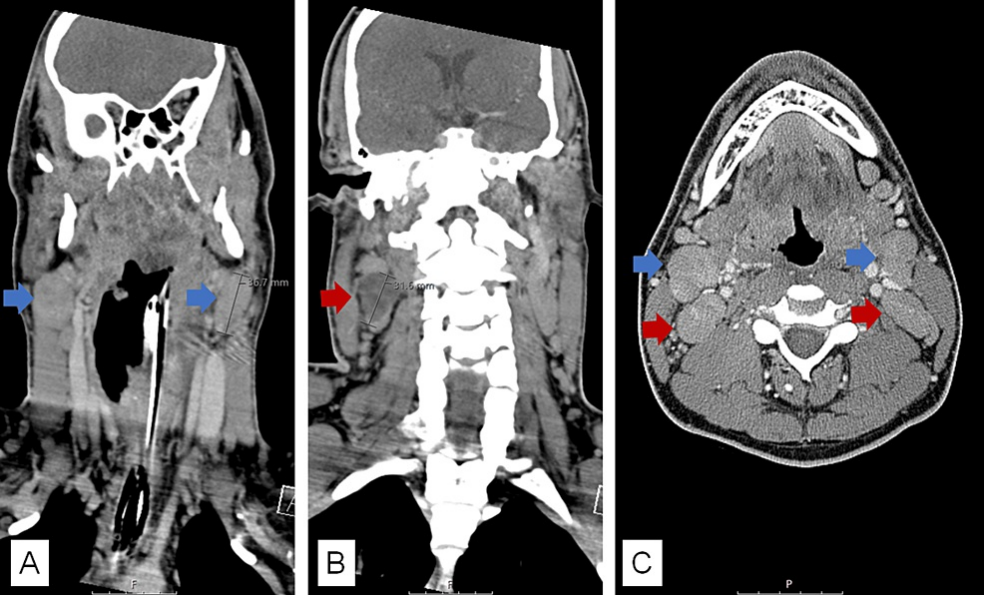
Neck CT scan coronal (A and B) and axial (C) views (A) Level 2A: bilateral cervical lymphadenopathies (blue arrows). (B) Level 2B: necrotic right cervical lymph node (red arrow). (C) Level 2A (blue arrows) and 2B (red arrows) cervical lymphadenopathies.
